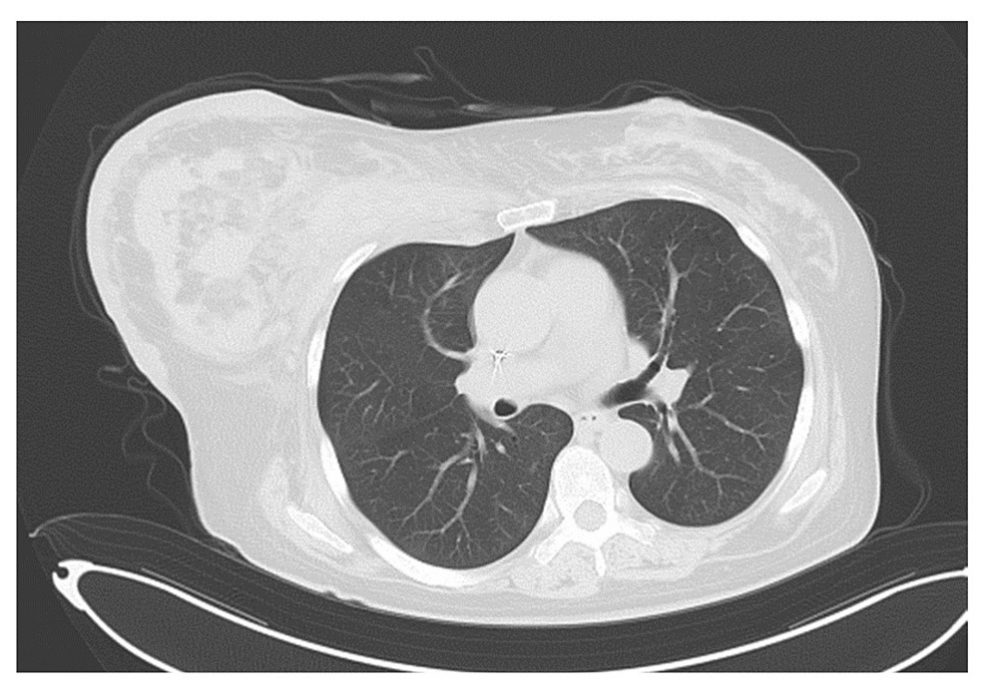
CT image of the patient on day 256. The ground-glass opacities previously identified on computed tomography at day 247 are no longer apparent.
